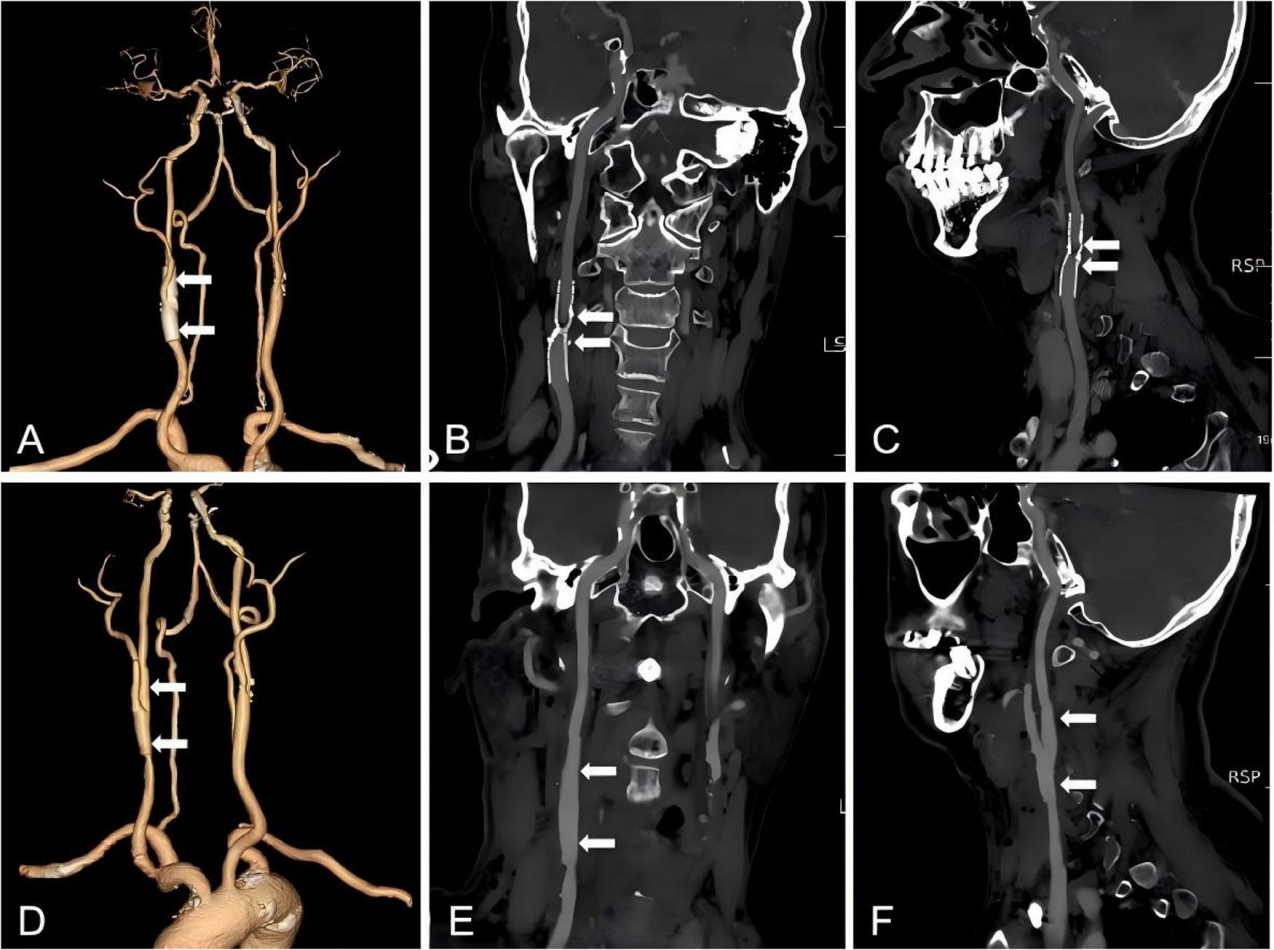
CTA images before and after the first CEA. Three-dimensional CTA images prior to CEA, and white arrows point to the location of the stent. (A) Coronal and sagittal CTA views show preoperative in-stent restenosis in the right internal carotid artery (white arrows). (B–C). Three-dimensional CTA images following CEA combined with stent removal, and white arrows point to the site of stent excision. (D) Coronal and sagittal CTA sections show resolution of restenosis after operation (white arrows). (E–F).
